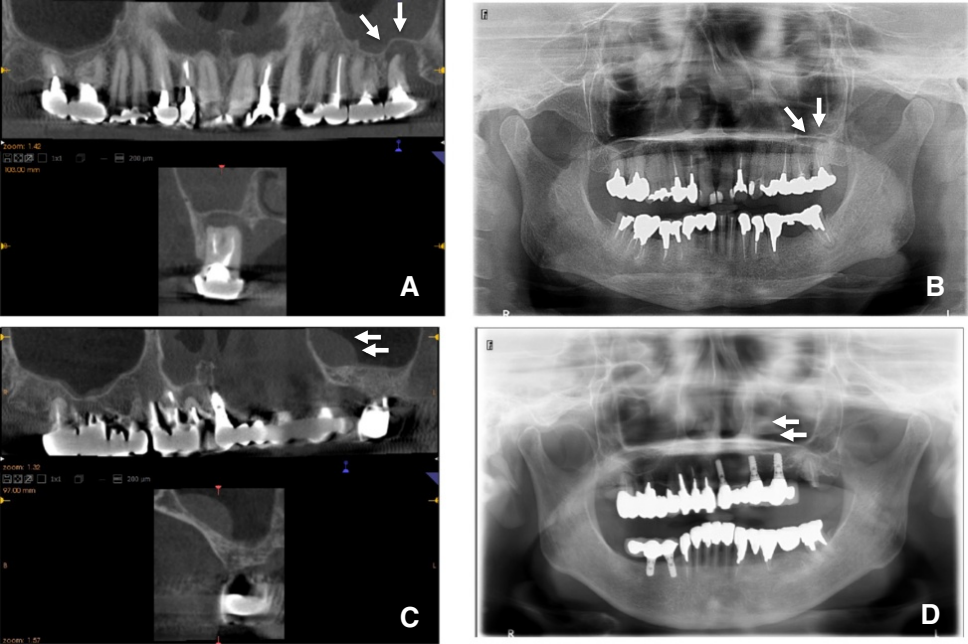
Images of mucosal thickening in the maxillary sinus. The CBCT (A) and panoramic radiograph (B) of a 58-year-old female with mucosal thickening >3 mm undergoing preoperative planning for implantation of a right maxillary molar. The CBCT (C) and panoramic radiograph (D) of a 66-year-old female with mucosal thickening <3 mm undergoing preoperative planning for implantation of a left maxillary molar. The mucosal thickening is clearly visualized on CBCT (A and C). However, the lesion >3 mm is visualized on panoramic radiographs (B), but that <3 mm is not (D).
